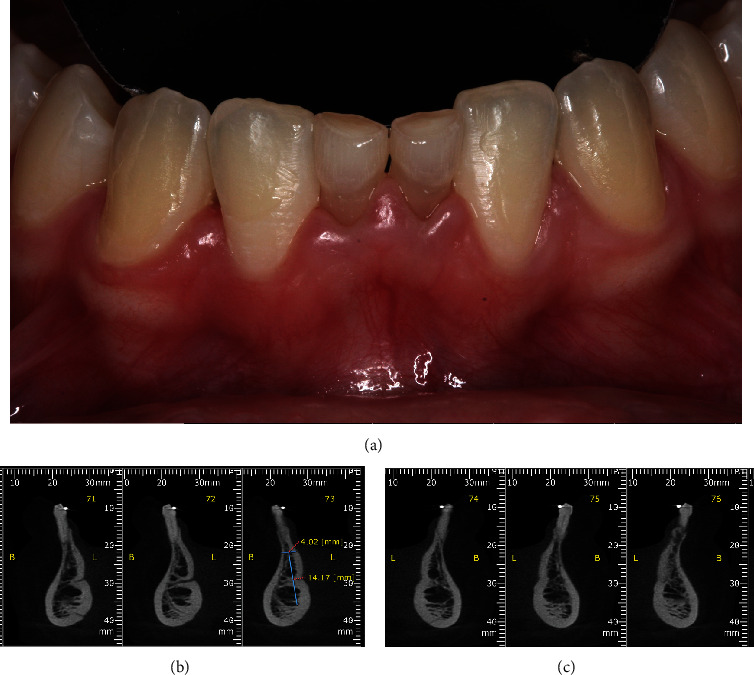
(a) Clinical image of the lower anterior region showing the presence of deciduous central incisors in the mandible with limited space for replacement of both teeth with dental implants. CBCT scan revealed a bone height of 14.17 mm and width of 4.02 mm in the anterior mandibular region; (b) a bone height of 13.42 mm; (c) width of 4.48 mm in the right upper lateral incisor; and a bone height of 14.50 mm and width of 5.70 mm in the left upper lateral incisor.
